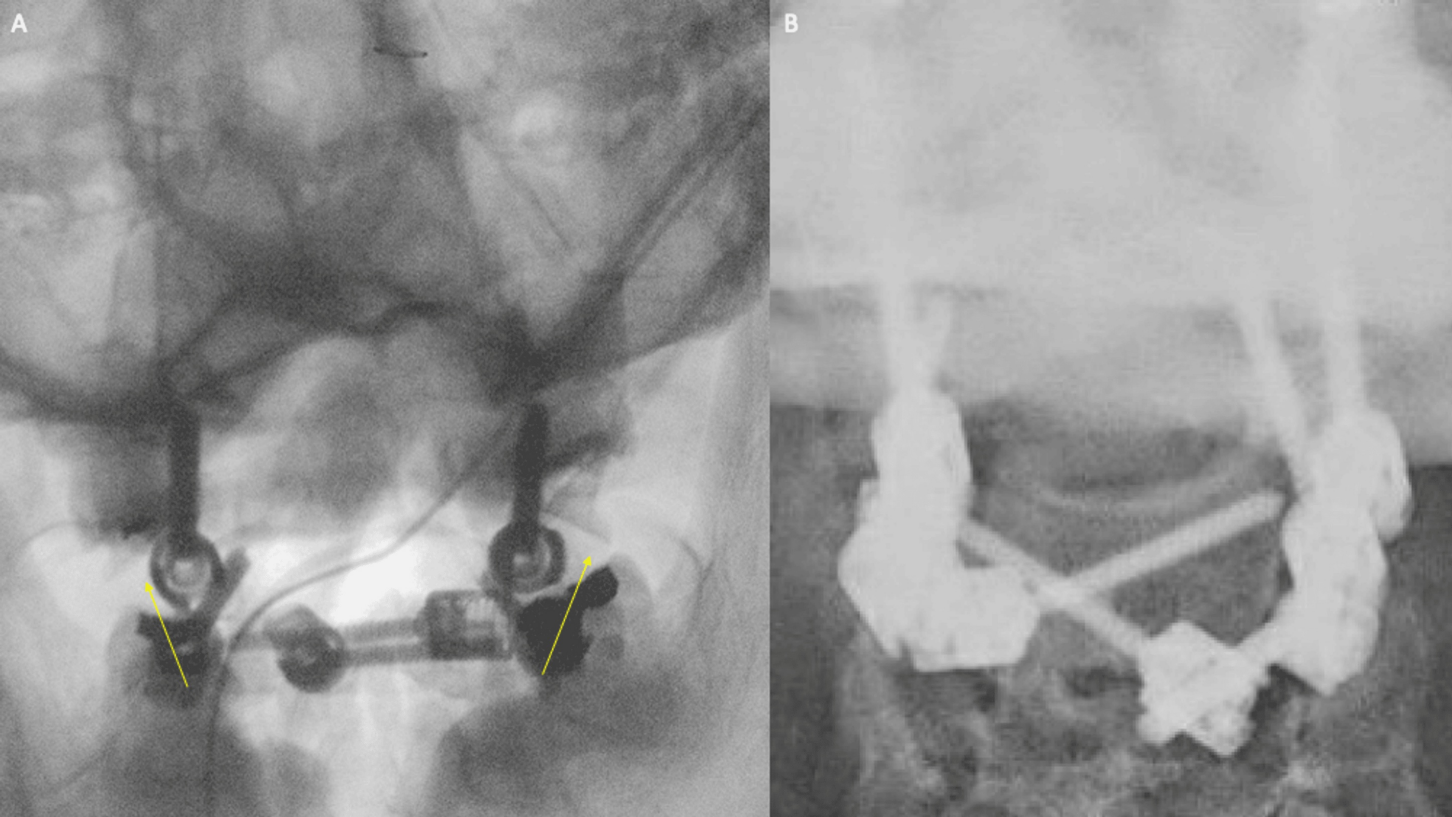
(A) Intraoperative X-ray after instrumentation shows significantly increased C1 lateral mass displacement compared to preoperative imaging (yellow arrows). (B) Post-reduction shows a connected construct with improved C1-2 lateral mass alignment.
